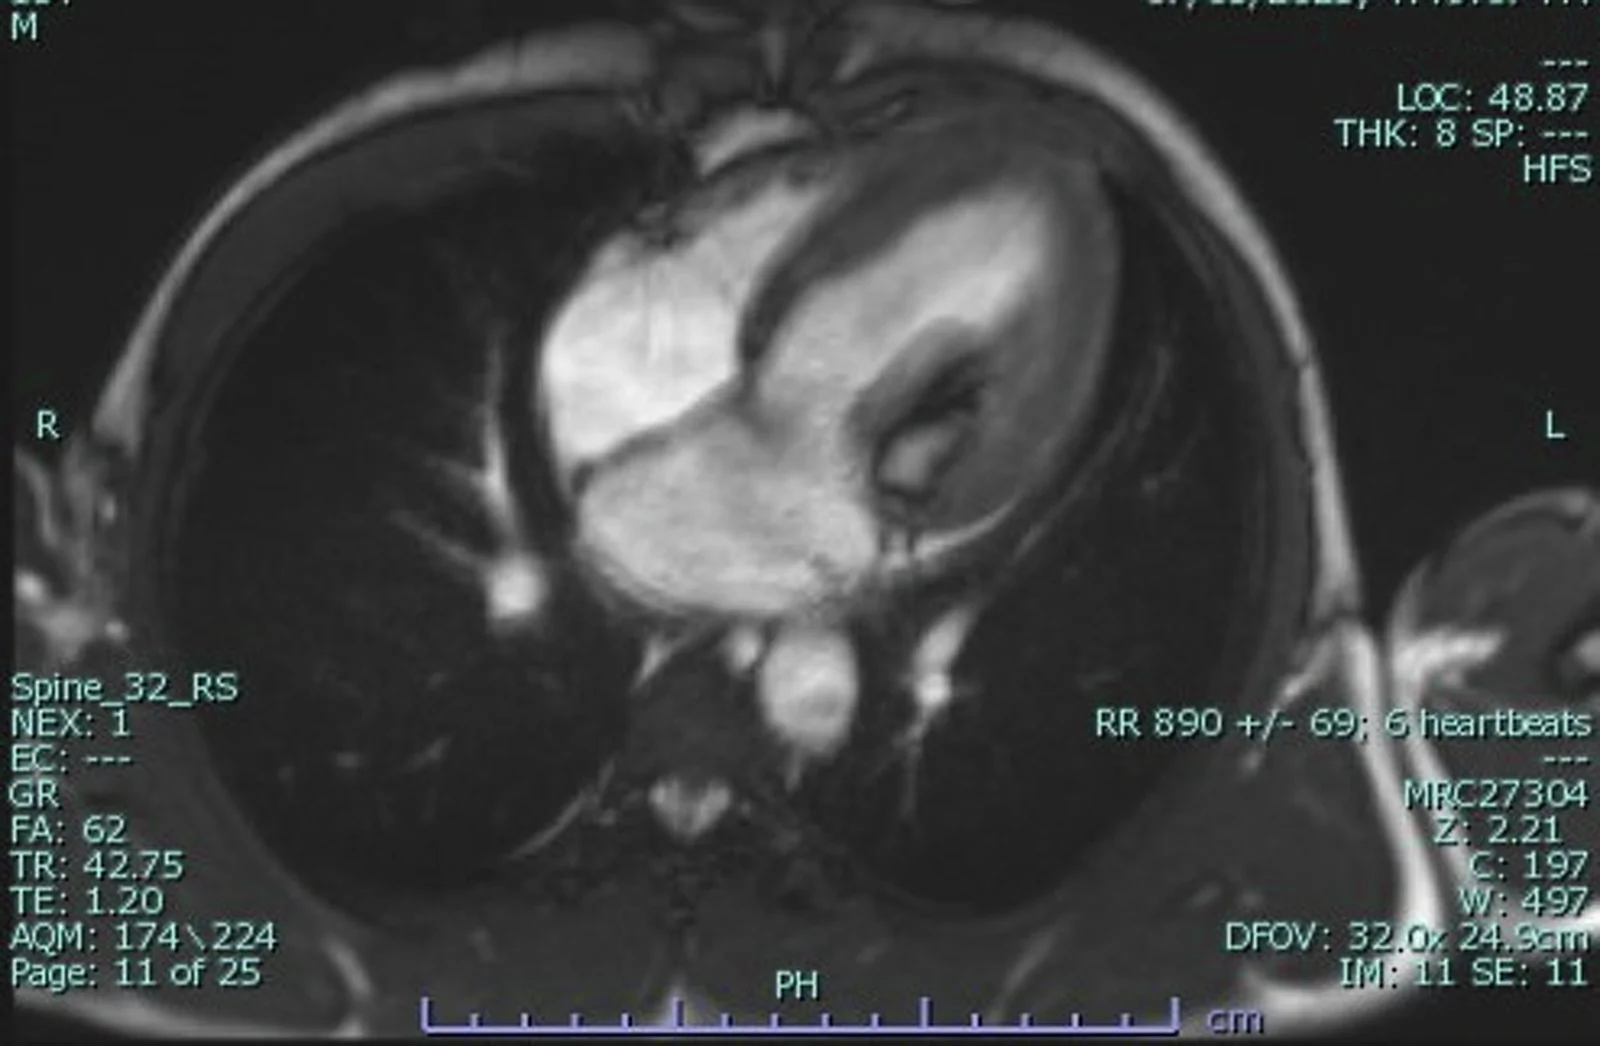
Cardiac Magnetic Resonance Imaging Showing Left Ventricular Outflow Tract Mass Extending into the Basal Anterior Wall of the Left Ventricle, Showing Homogeneous Late Gadolinium Enhancement AQM = acquisition matrix; DFOV = display field of view; EC = echo collection/echo count; FA = flip angle; GR = gradient recall (gradient echo); IM = image; LOC = location/localizer; NEX = number of excitations; PH = potential of hydrogen; RR = reconstruction radius/reconstruction region; TE = echo time; THK = slice thickness; TR = repetition time.
